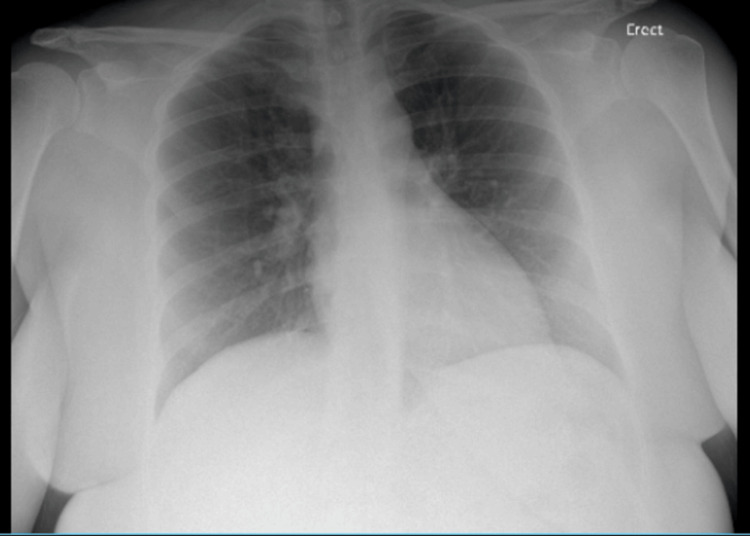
Initial chest X-ray on day one of admission was unremarkable
